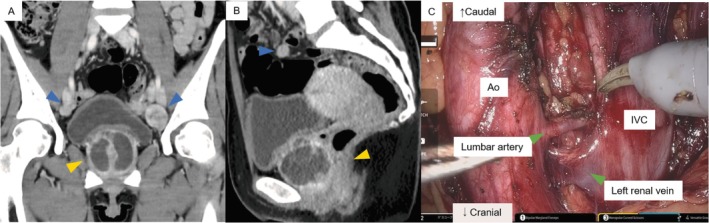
Sagittal (A) and coronal (B) contrast‐enhanced computed tomography images of Case 2 preoperatively. The yellow arrows indicate a 45 mm × 42 mm × 48 mm cystic tumor in the urethra, and the blue arrows indicate bilateral obturator and common‐iliac lymph node enlargement. (C) A super‐extended lymph node dissection, including para‐aortic lymph node, was performed. The green arrows indicate the lumbar artery and the left renal vein, respectively. Ao, aorta; IVC, inferior vena cava.
